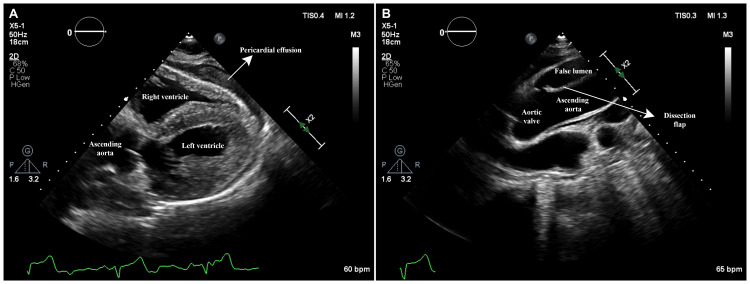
Ascending aortic dissection with cardiac tamponade Transthoracic echocardiogram in a patient with ascending aortic dissection and cardiac tamponade: (A) Subcostal view showing a large pericardial effusion causing RV collapse. (B) A high-right parasternal view demonstrating the intimal dissection flap in the ascending aorta. RV: right ventricular Image credit: Dinkar Bhasin
